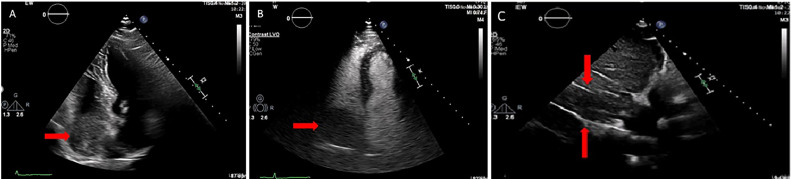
(A) Transthoracic echocardiography revealed a large echogenic mass in the right atrium (red arrow), (B) Contrast echocardiography showed that the mass in the Fig. 1A does not enhance with intravenous contrast agent, indicating that this mass may be a bland thrombus (red arrow), (C) Inferior vena cava appears distended and occluded with echogenic material and no signs of flow (between the red arrows) in the transthoracic echocardiogram.
